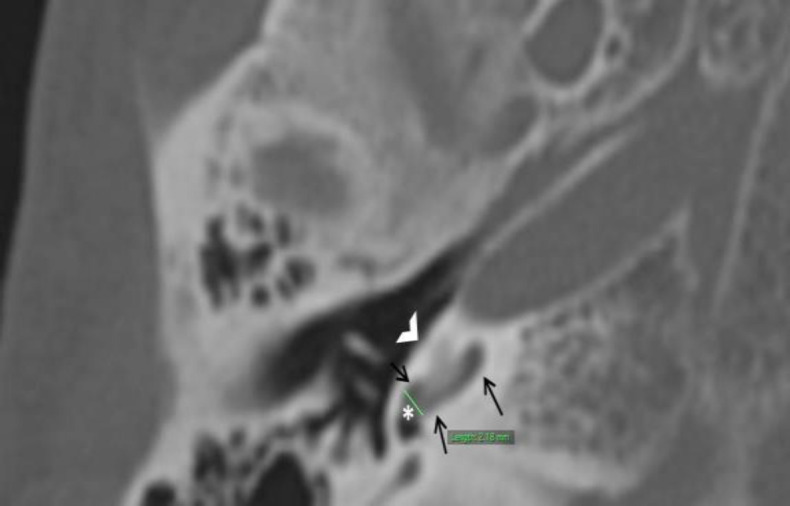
Axial CT image of temporal bone demonstrating method used to measure diameter of basal turn of cochlea (BD) as depicted by straight green line. Note that the measurement is done adjacent to the round window (asterisk). Basal turn of cochlea is denoted by black arrows while white open arrow heads indicate promontory
